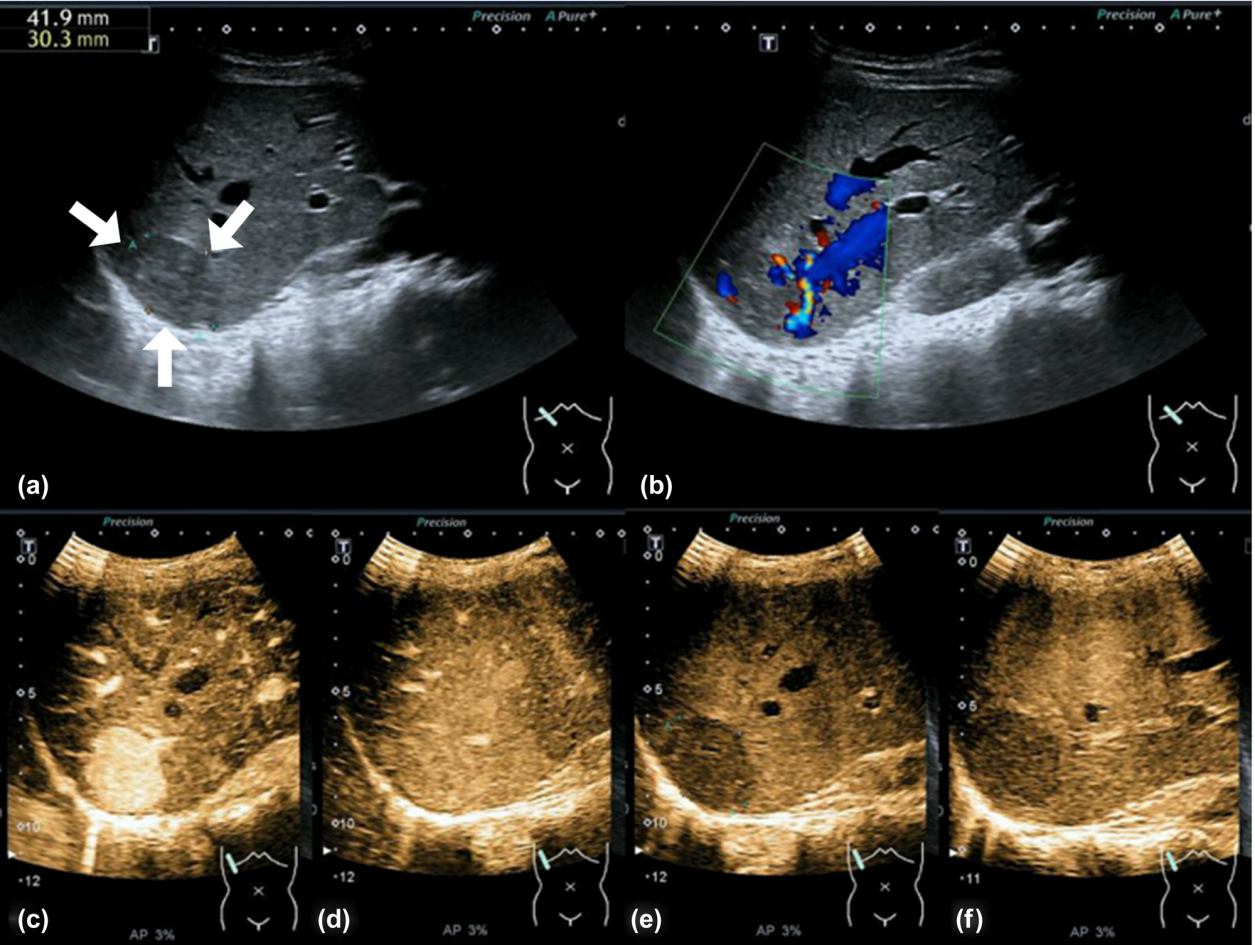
Abdominal ultrasonography and Sonazoid contrast-enhanced ultrasound images (Sonazoid 0.5 mL bolus injection, Toshiba Aplio 500, and 3.75 MHz convex array probe) of a primary hepatic perivascular epithelioid cell tumor. (a) A heterogeneous hypoechoic nodule is demonstrated on B-mode ultrasound (arrows). (b) Color Doppler flow images reveal abundant blood flow at the tumor margins. (c–f) Sonazoid contrast-enhanced ultrasound in the arterial (c, 23 s), portal (d, 100 s), equilibrium (e, 5 min), and post-vascular (f, 10 min) phases. Hyper-enhancement is observed in the arterial phase, with iso-enhancement in the portal phase and mild hypo-enhancement in the equilibrium and post-vascular phases.
